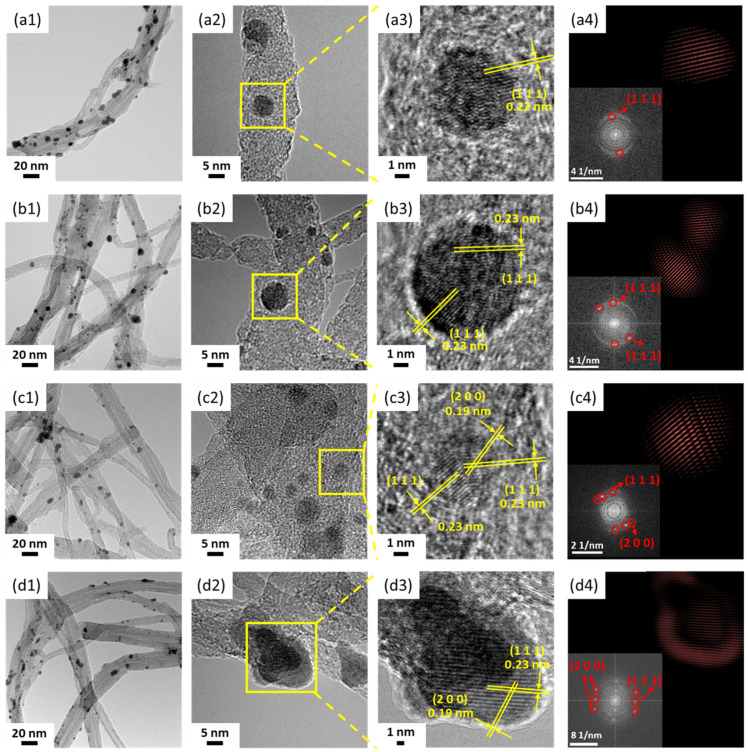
TEM images, fast Fourier-transform (FFT), and inverse FFT patterns of Pd/A-CNT after synchrotron X-ray irradiation times of 0.5 min (a1–a4), 1 min (b1–b4), 2 min (c1–c4), and 4 min (d1–d4). The FFT patterns are shown as the inserted figures in (a4–d4).
